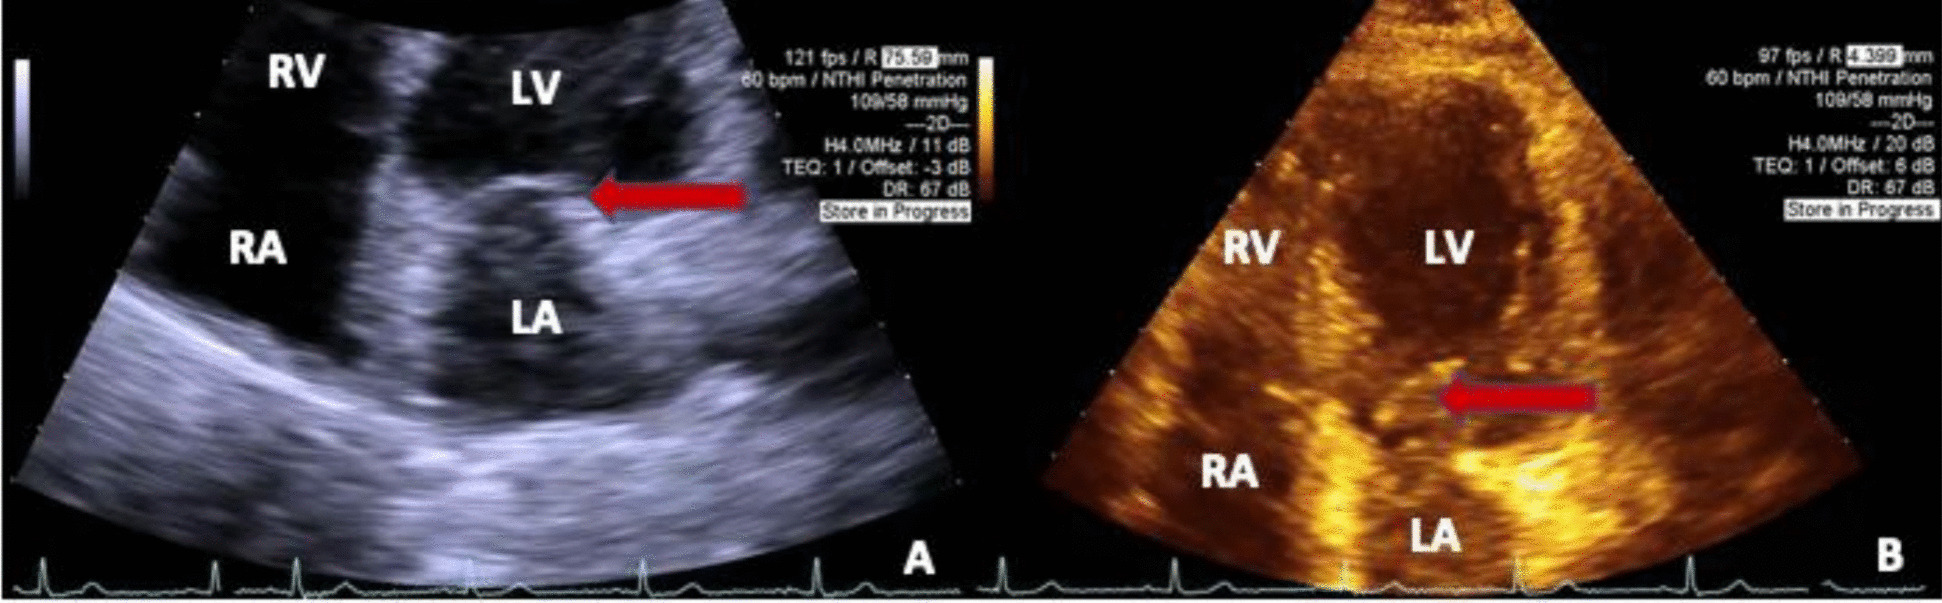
Transthoracic echocardiography images from post hospital follow up. A An apical four chamber view of the heart with the left atrium, left ventricle, right atrium, right ventricle, and the mitral valve indicated by the red arrow. B Another apical four chamber view with the same labels, again with the mitral valve indicated by the red arrow. The two intra-atrial masses are no longer visualized
